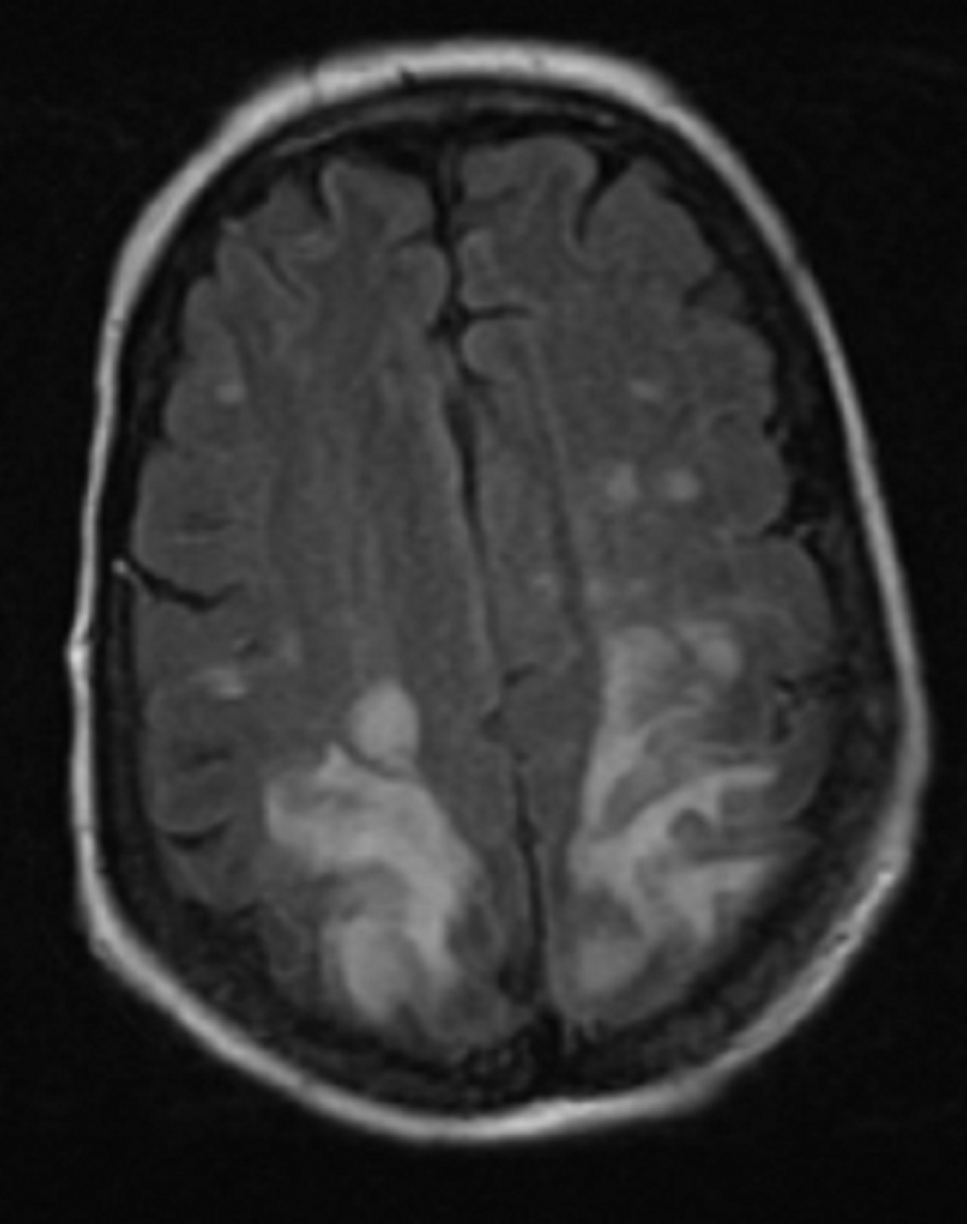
MRI brain showed T2/FLAIR signal hyperintensity over the bilateral posterior head regions, consistent with PRES
